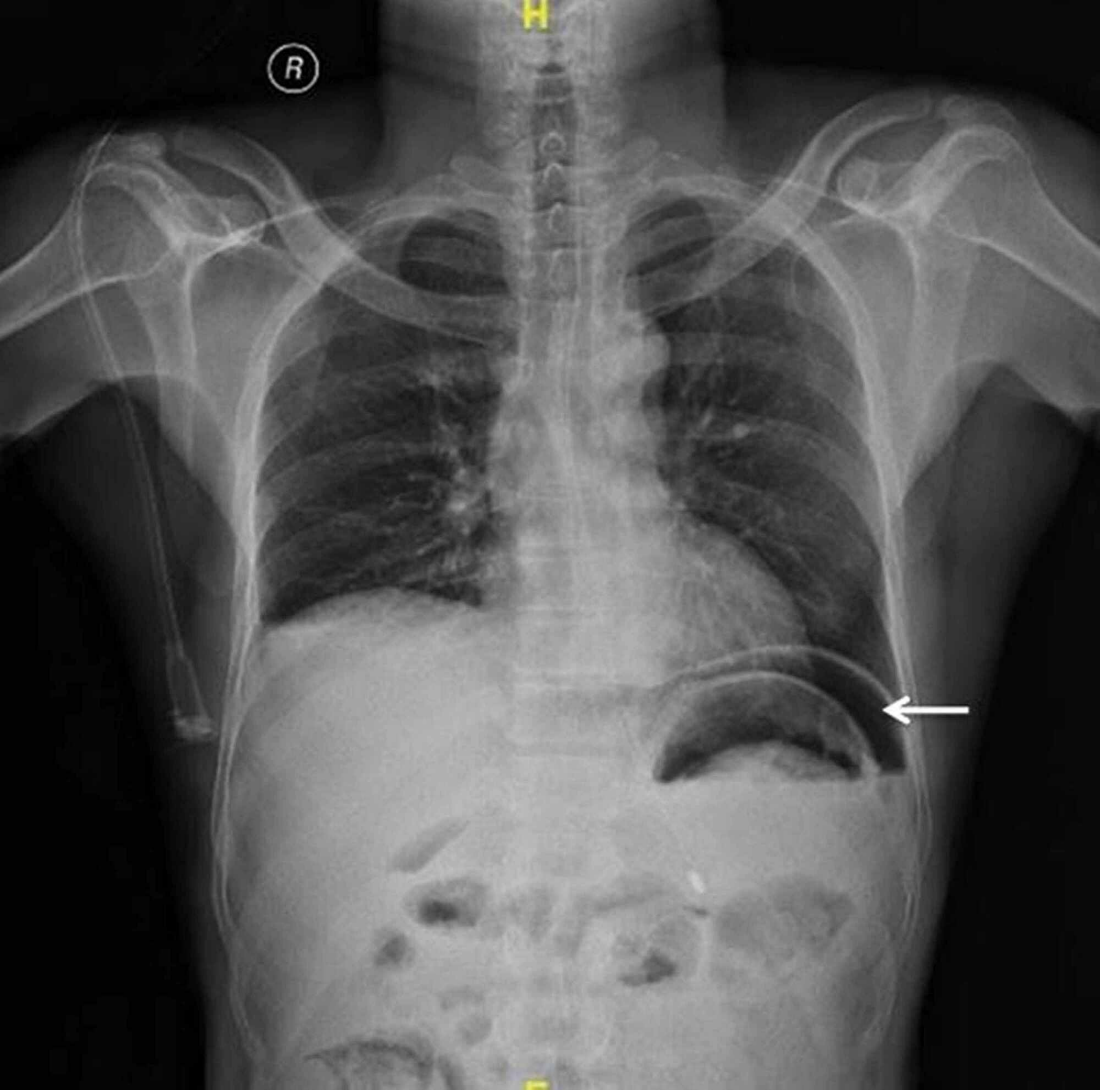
X-ray of the chest and abdomen showing gas under the diaphragm displacing fundus of the stomach.
